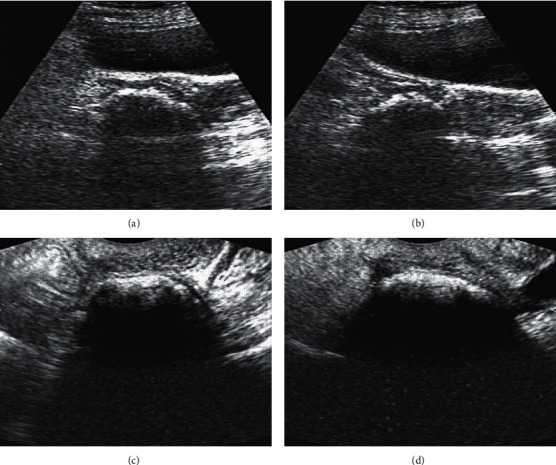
Postoperative ultrasound images of a 42-year-old patient undergoing total hysterectomy. ((a), (b)) Pelvic cavities; ((c), (d)) transvaginal uterus.
